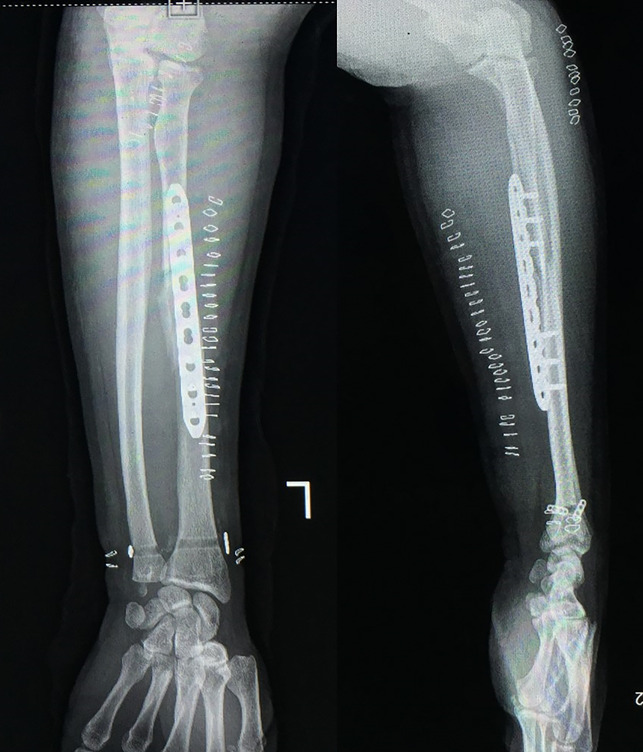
Postoperative radiographs showing anteroposterior and lateral views.
